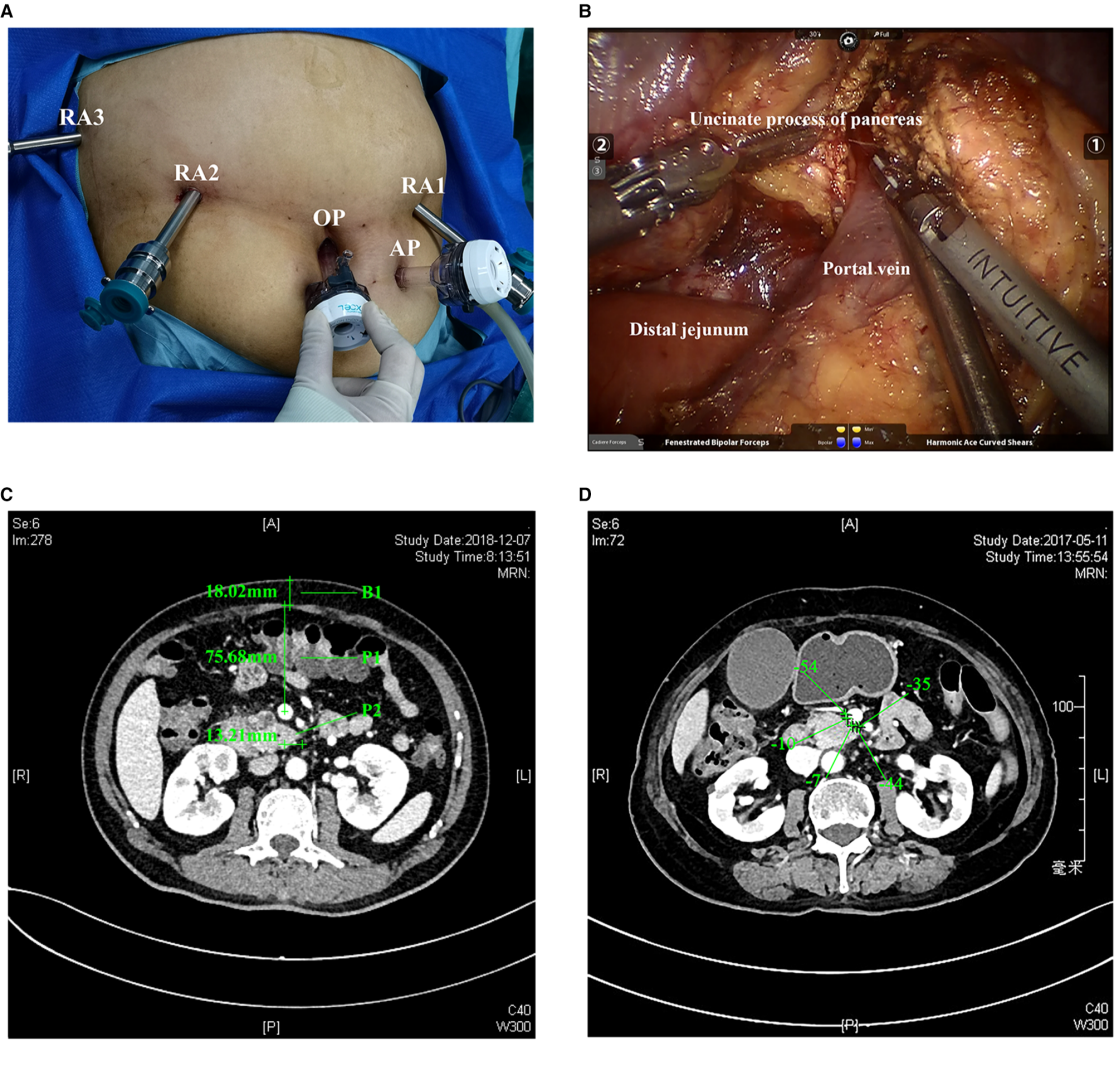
(A). Placement of the five ports. RA1, RA2, RA3, the OP, and AP. (B). Separation of the uncinate process of the pancreas and portal vein. OP, optic port; AP, assistant port. (C). Actual measured P1, P2, and B1 indexes. (D). Peripancreatic fat density. The density value is the average of the density where the five plus signs are located in the figure. The CT image is in the venous phase. CT, computed tomography; P1, mesenteric tissue thickness; P2, length of the uncinate process; B1, thickness of the abdominal wall.
